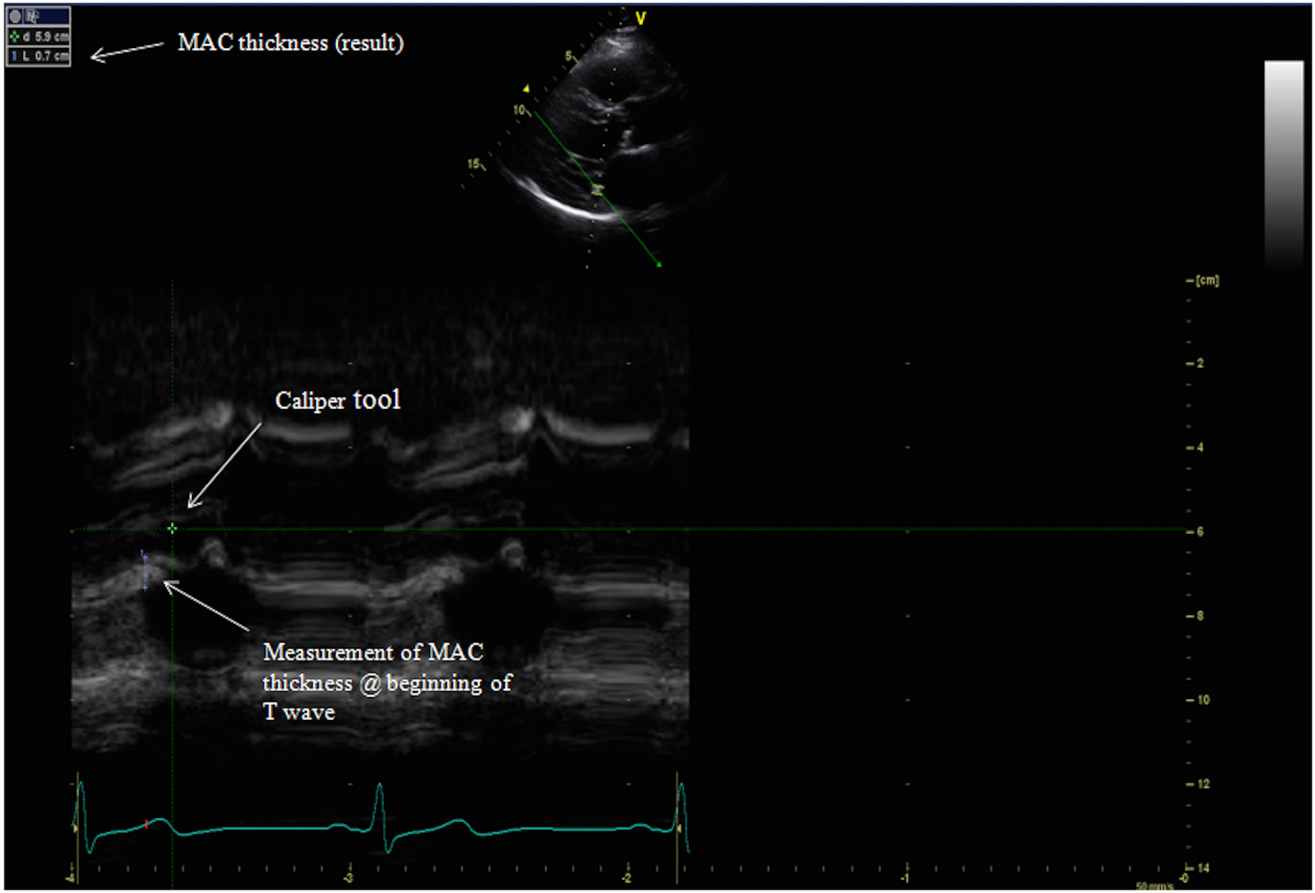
M-mode measurement of MAC in the parasternal long axis view. Abbreviations: Mitral Annular Calcification (MAC).
